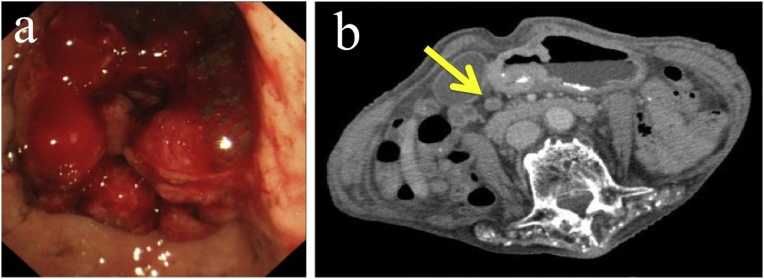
(a) Upper gastrointestinal endoscopy showed a Borrmann type3 tumor with pyloric stenosis and bleeding. (b) Contrast-enhanced computed tomography scan showed lymph node involvement around the stomach but no obvious distant metastases.
